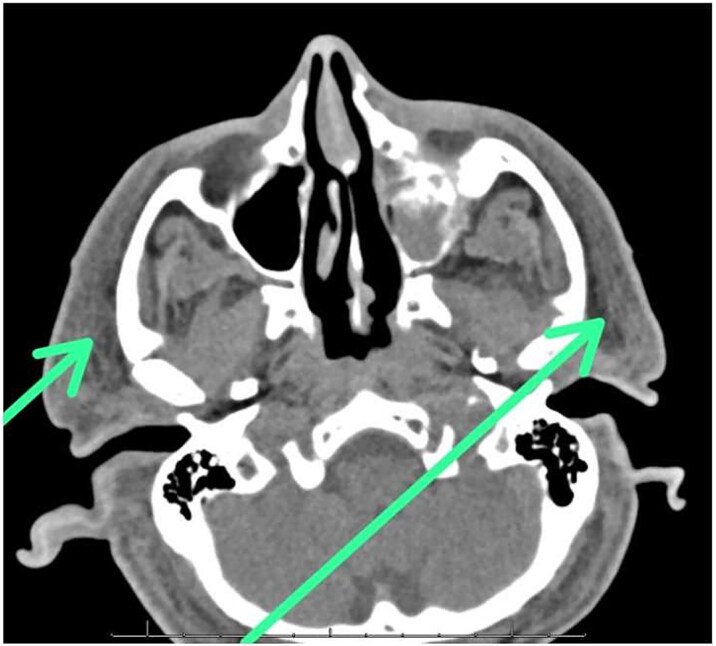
Axial CT image showing subcutaneous fat swelling in the face, most prominently involving the right external ear. Asymmetrical soft tissue thickening is also noted along the right ear region.
